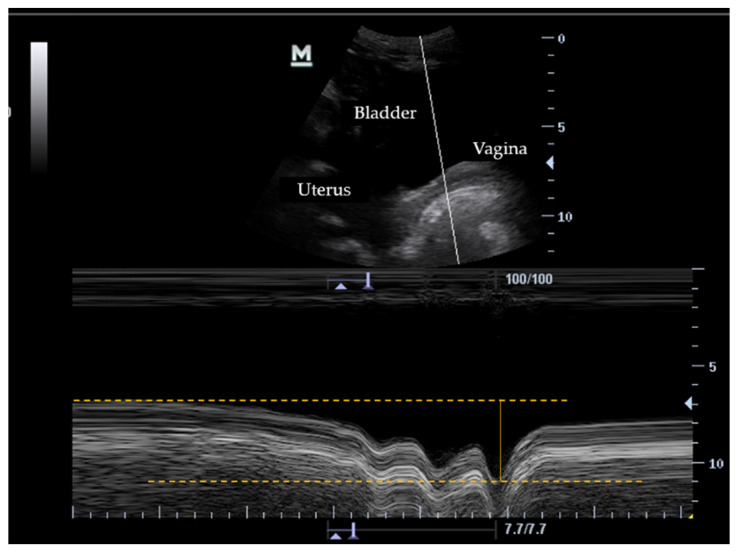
Ultrasound measurements of the bladder base displacement during a cough in sagittal plane. In the top of the figure pelvic structures are shown. Below is represented the timeline of M mode. The dotted horizontal lines represent the bladder base in rest position and the position during a cough. The vertical continued line shows the bladder displacement.
